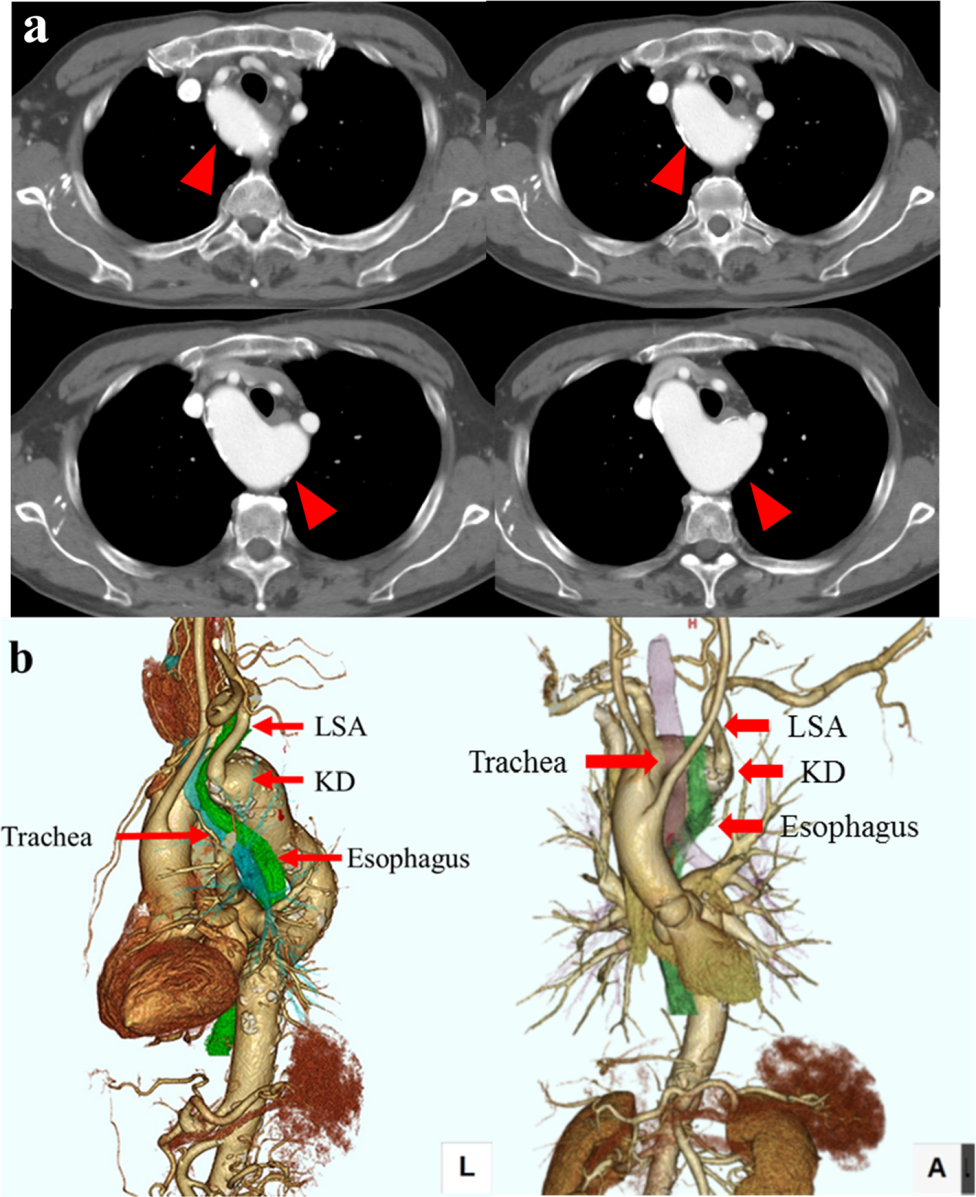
Preoperative CT findings. a Transverse image: the right aortic arch (arrow) surrounding the trachea and esophagus from the dorsal side. b 3D-CT findings: Edwards classification type IIIB1, with Kommerell’s diverticulum at the origin of the descending aorta, from which the left subclavian artery can be seen to be bifurcating ectopically. LSA: left subclavian artery, KD: Kommerell’s diverticulum
